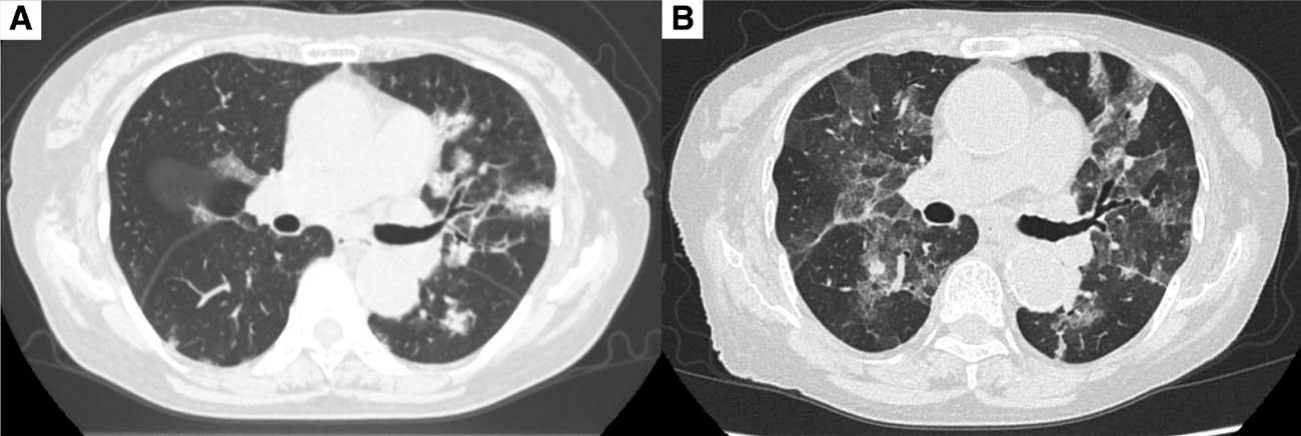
(A) Chest computed tomography image taken approximately 20 years ago, showing multiple patchy areas in both lungs. (B) After vaccination, image findings were similar to those 20 years ago.
